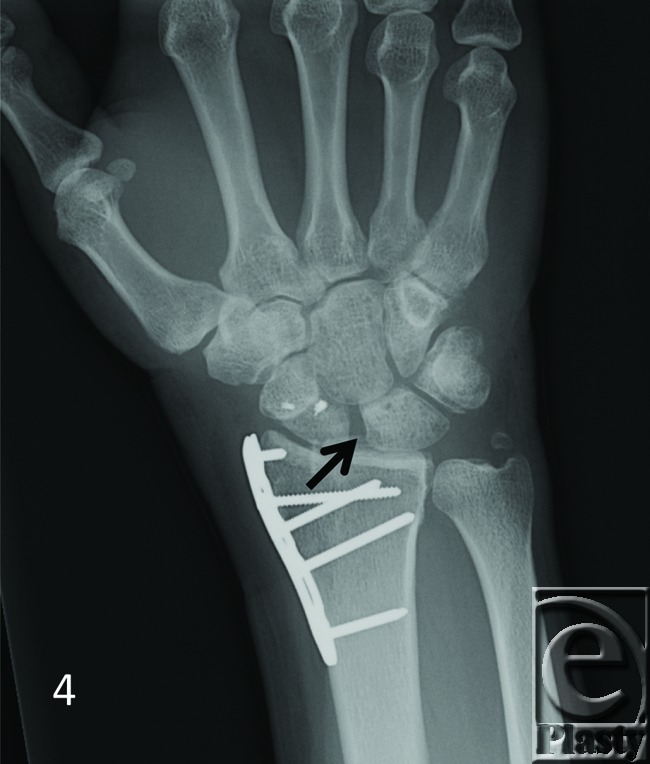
A 30-year-old man with prior ORIF and SLIL ligament repair. Notice mild gaping of S-L interval (*arrow*) on conventional posteroanterior X-ray view. ORIF indicates open reduction internal fixation; SLIL, scapholunate interosseus ligament.
